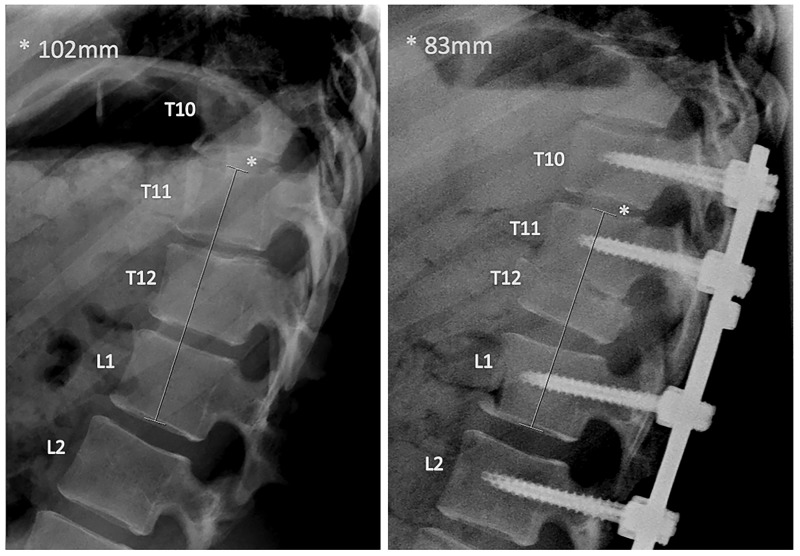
Pre- and Postoperative Lateral Radiograph (left and right, respectively) The preoperative thoracolumbar lateral radiograph on the left demonstrates the initial spinal column length in millimeters. The postoperative thoracolumbar lateral radiograph on the right demonstrates partial T12 corpectomy, with associated pedicle, lamina, and facet removal, including instrumentation. Nineteen millimeters of overall spinal column shortening was achieved.
